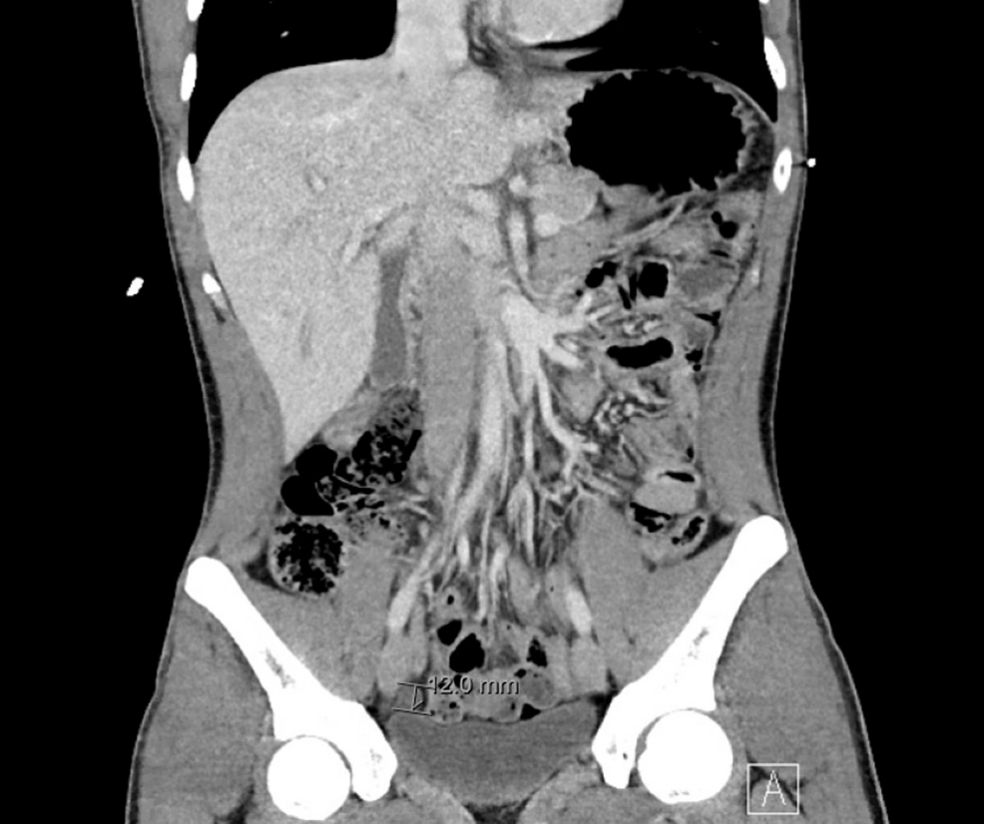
CT scan of the abdomen showing dilated appendix with fluid-filled lumen along with intraluminal appendicolith suggestive of appendicitis.
